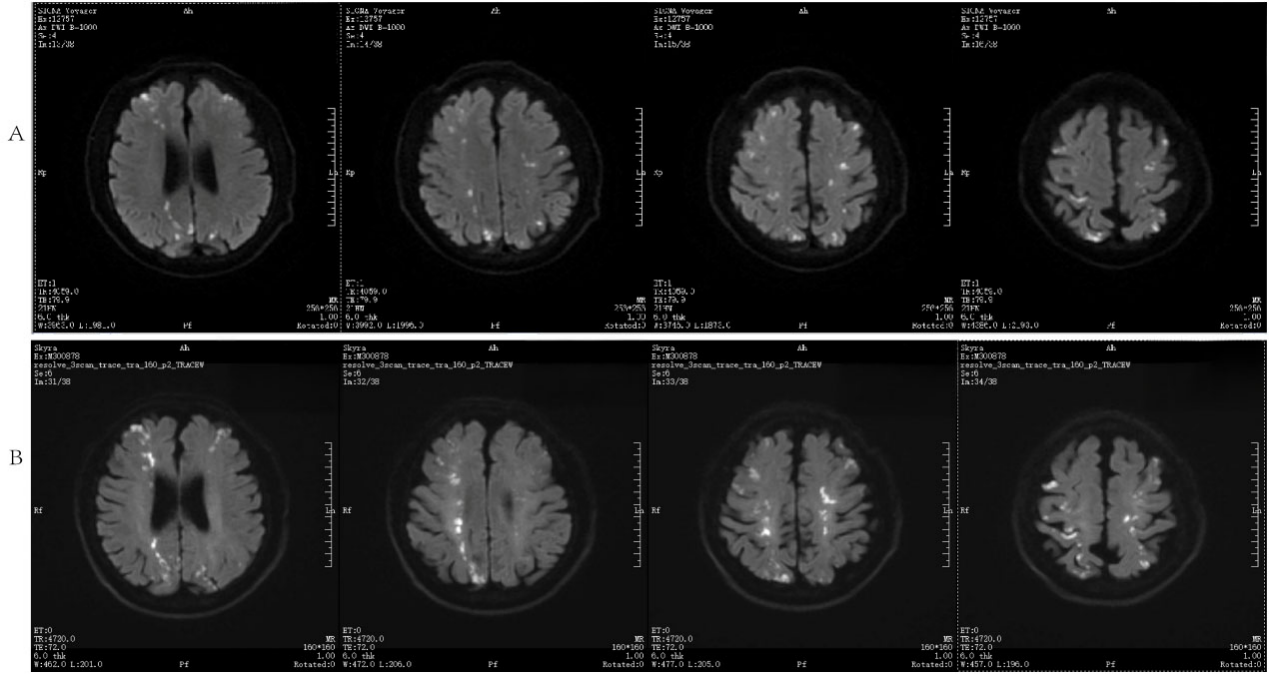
DWI and cranial MRI (A) inspected on March 27, 2025, (B) analyzed on April 1, 2025.
